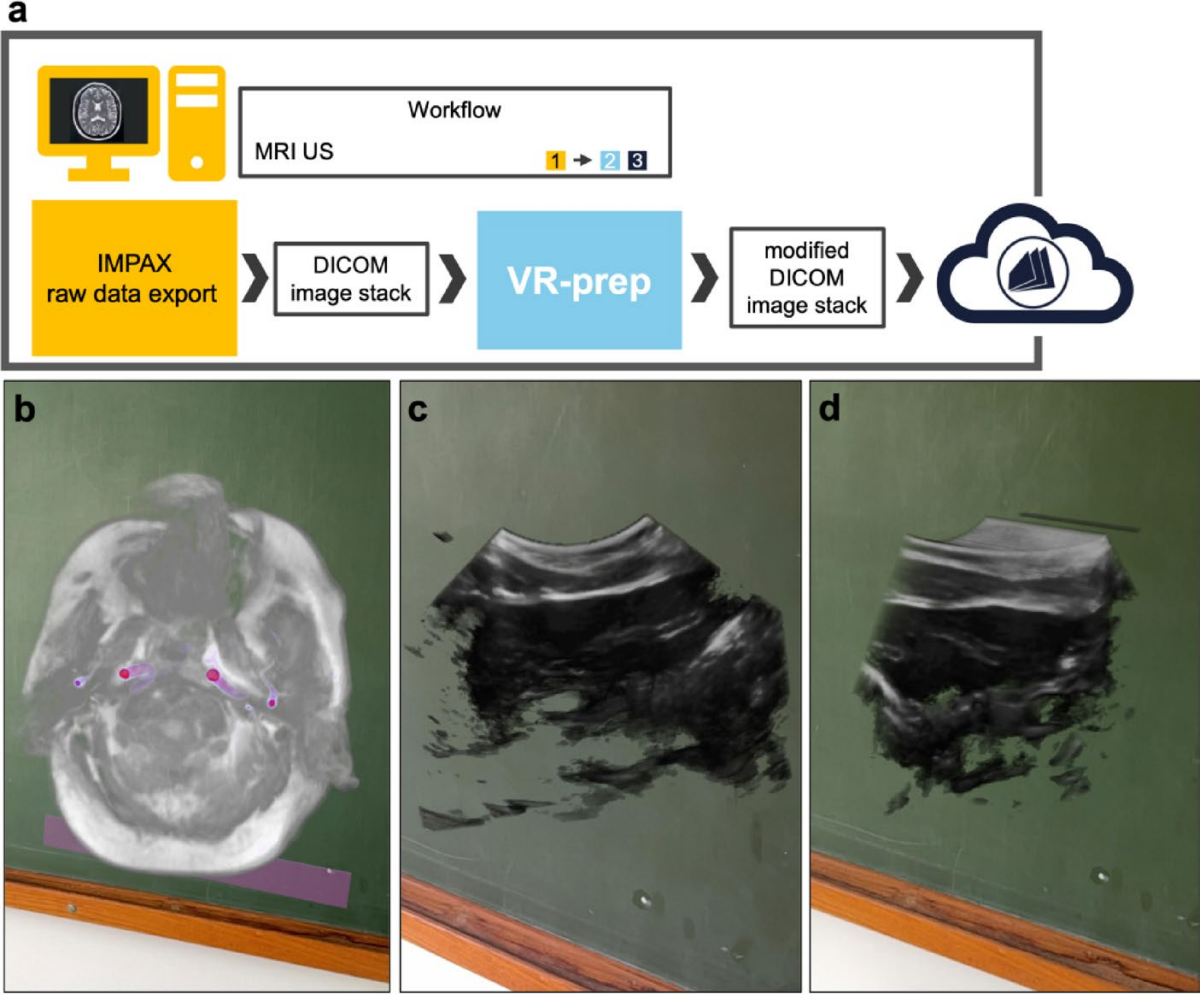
Workflow for the visualization of MRI and ultrasound data with VR-prep and Medical Imaging XR. (a) Demonstration of incorporation of VR-prep in the workflow. IMPAX as synonym for Picture Archiving System. (b) AR image displays an MRI angiography of the head/neck region (grayscale) enhanced by contrast media (purple). (c-d) The AR images display an ultrasound image stack at two different orientations. Note that the AR image on the right side is cropped in real-time via Medical Imaging XR.
